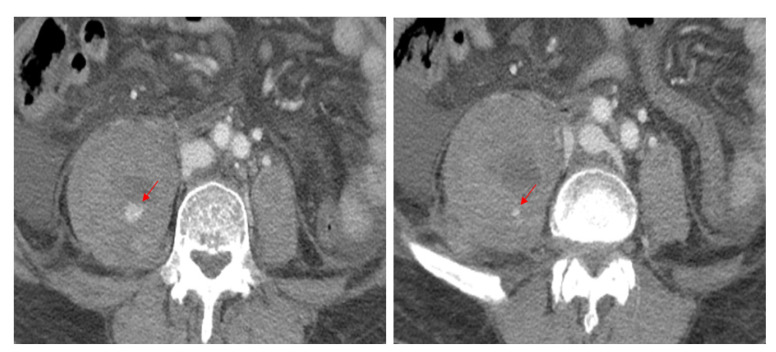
Contrast-enhanced CT scans reveal high attenuation, indicating active bleeding (arrows) from the right second and third lumbar arteries within the hematoma.
